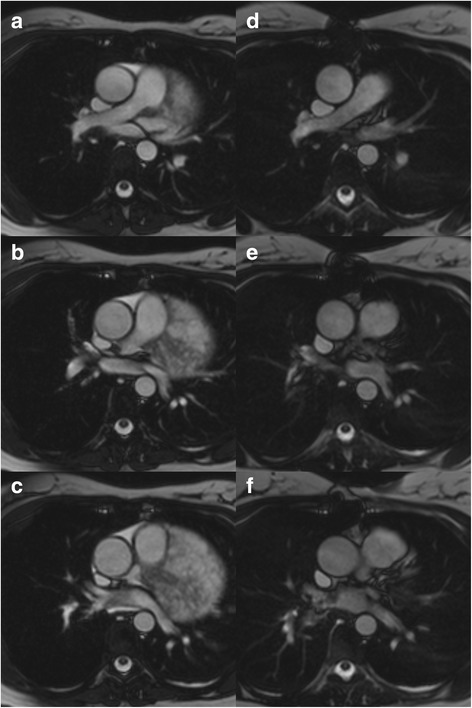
Cine movie MRI images in transverse planes in the diastolic phase before surgery (a-c) and at the three-year follow-up (d-f)
